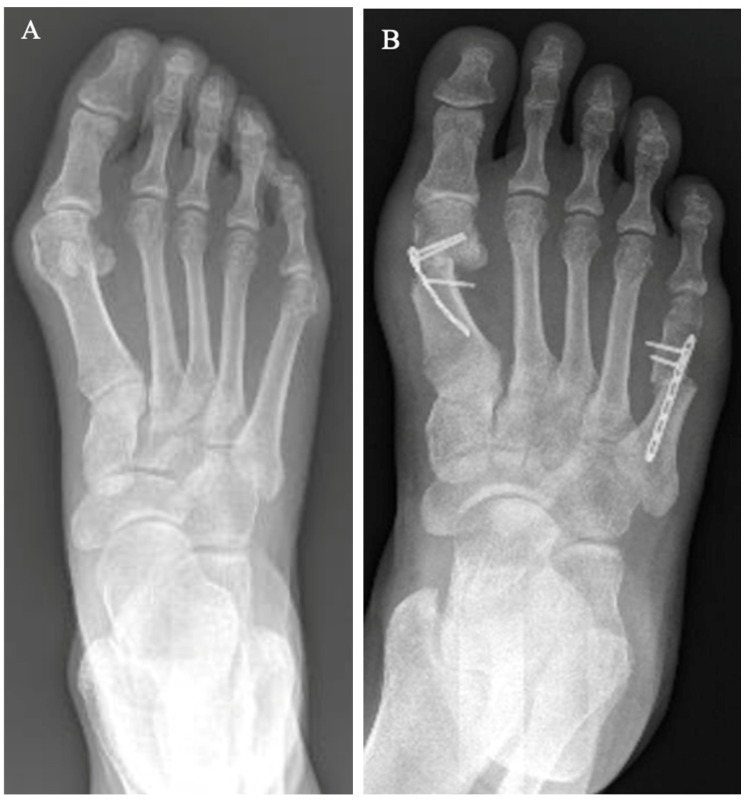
Weight-bearing anteroposterior radiographs of a 45-year-old female patient with symptomatic Coughlin type III bunionette deformity. (A) Preoperative radiograph showing lateral prominence of the fifth metatarsal head and increased fifth metatarsophalangeal and fourth-fifth intermetatarsal angles. (B) Postoperative radiograph obtained after mini-open fifth metatarsal osteotomy and intramedullary TRUE LOCK 2.0 mm Mini Plate fixation, demonstrating medial translation of the distal fifth metatarsal fragment, improved fifth ray alignment, and stable implant position.
